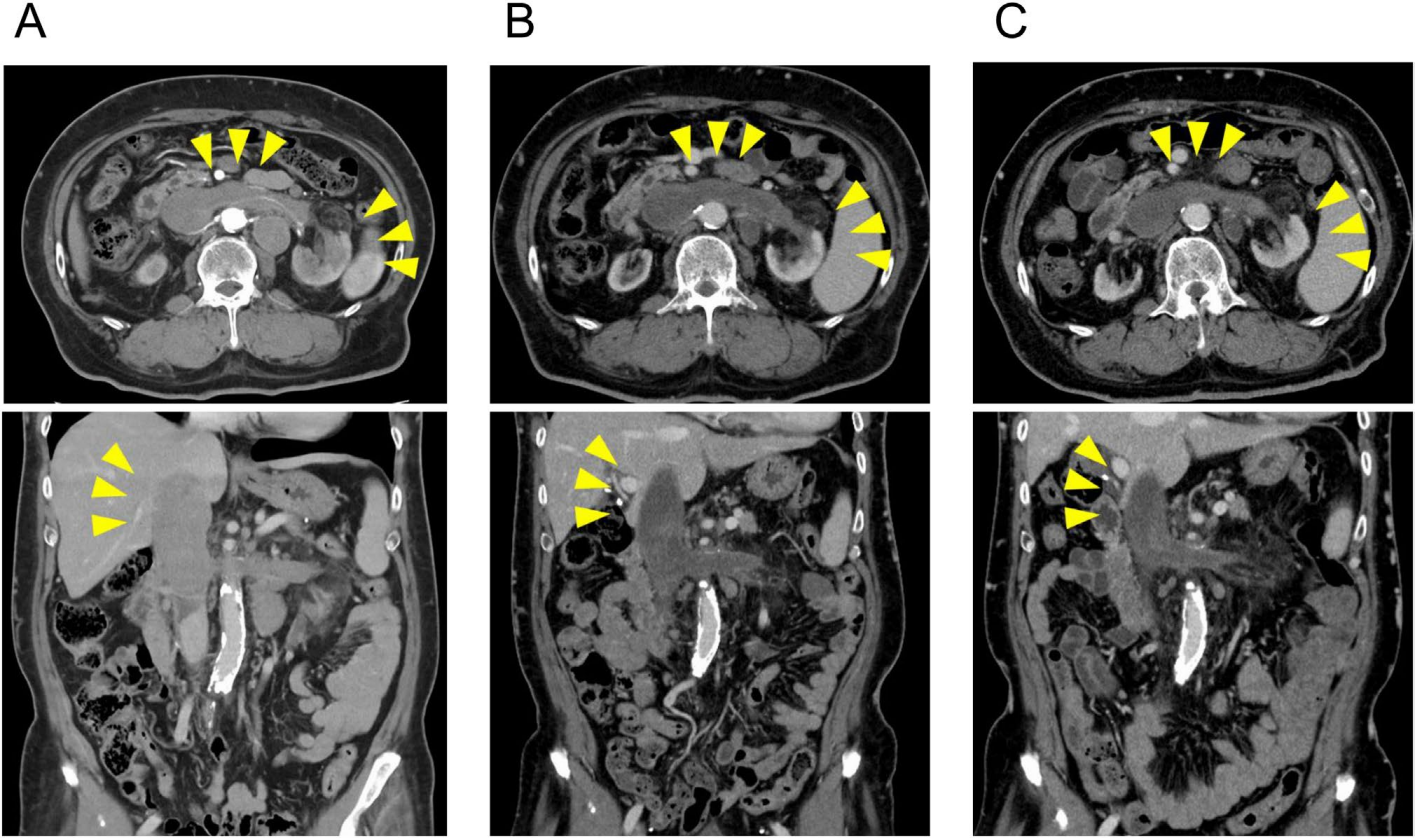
Dynamic computed tomography images of the primary kidney lesion: (A) before treatment with pembrolizumab plus lenvatinib, (B) 2 months after initiation of the combination therapy, and (C) 6 months after initiation of the combination therapy.
